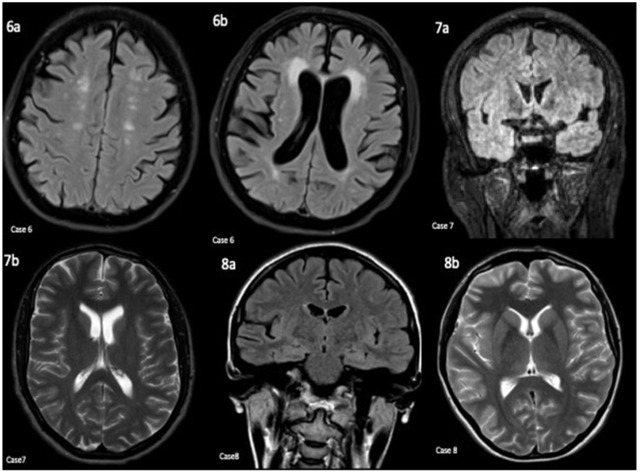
CASE 6: Axial FLAIR (6a,6b) sequences. Moderate to advanced generalized parenchymal volume loss without focal signal abnormality involving the mesial temporal lobes. There was also advanced white matter chronic microvascular ischemic changes. CASE 7: Coronal FLAIR (7a) and axial T2 sequence. Normal appearance of the mesial temporal lobes. The rest of the examination was also normal. CASE 8: Coronal & Axial FLAIR/T2 (8a,8b) sequence. Normal examination without any mesial temporal or other focal signal abnormalities.
